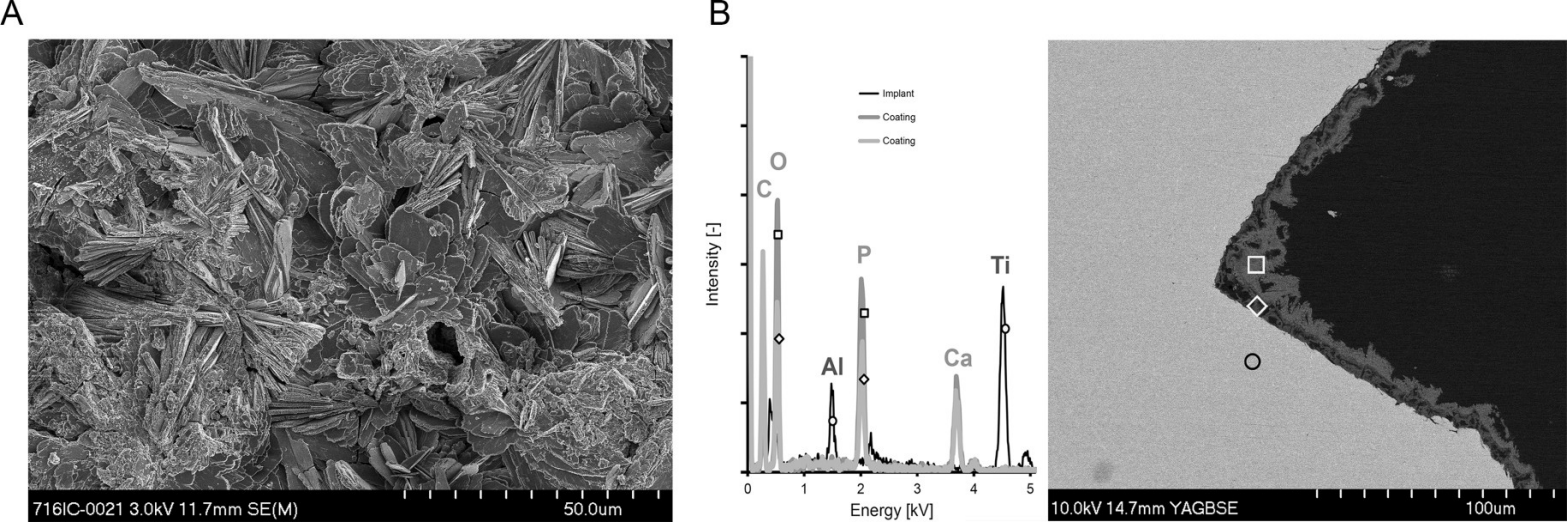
SEM analysis of surface morphology and coating thickness of the CaP coating used in the study. A.) Representative image of the CaP coating present on the TAN discs used during the *in vitro* aspect of the study. Scale bar is 50 μm. The image is from one disc and is representative of 2 further discs independently prepared and analyzed. B.) Left: Elemental composition of the CaP coating, as determined by energy dispersive X-ray analysis (EDX). The black line indicates the TAN screw, while the lower and upper layers of the coating are indicated by the light grey and dark grey lines, respectively. Right: Representative image of a longitudinal cross-section of the CaP-coated TAN screw (light grey) demonstrating the thickness of the CaP coating present on the screw and the regions of interest selected for the EDX analysis: screw–open black circle; lower level of CaP coating—open white diamond; upper layer of coating—open white square. Image is from one screw and is representative of 1 further screw, independently prepared and analyzed. Scale bar is 100 μm.
